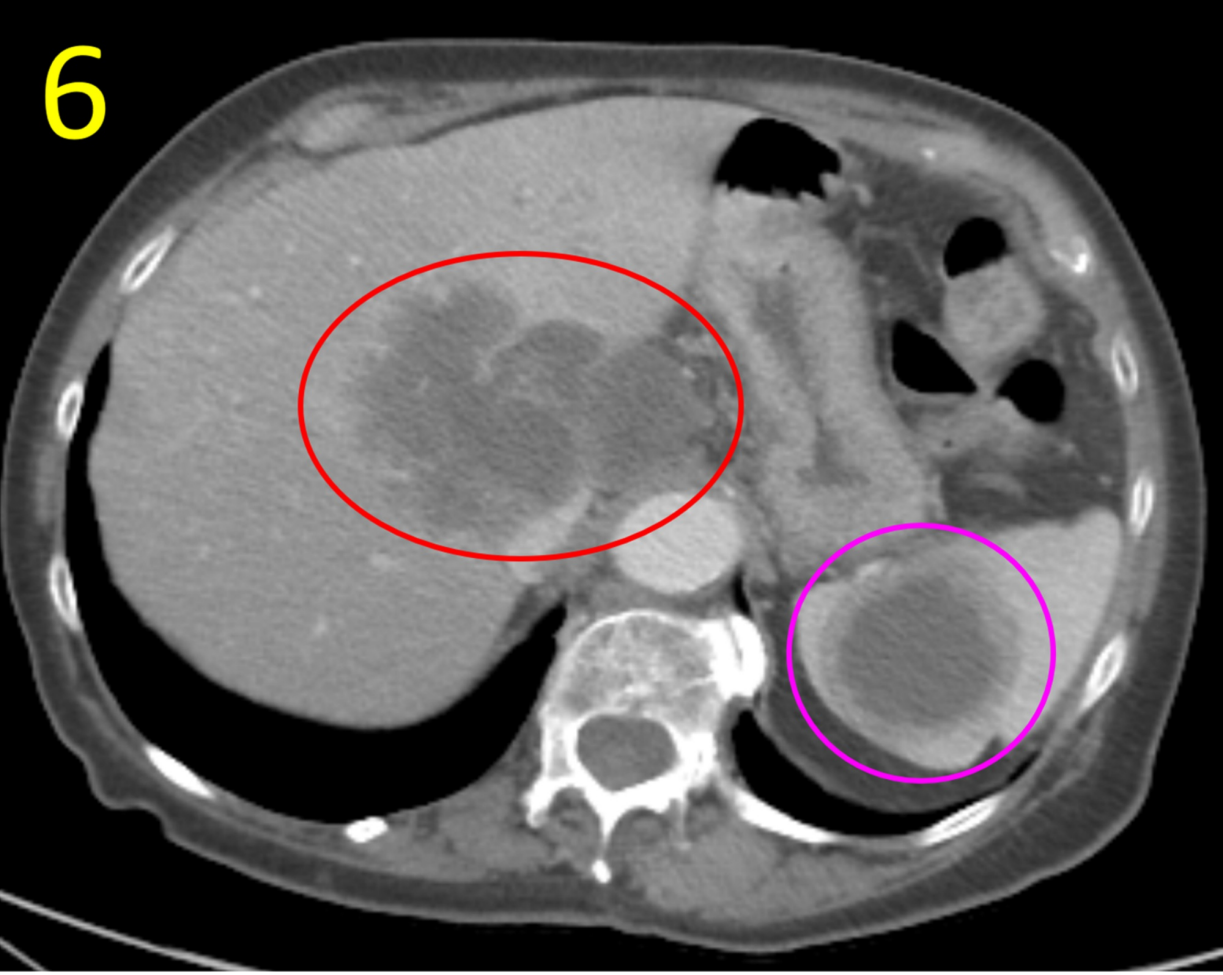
Abdominal CT Scan This abdominal CT scan demonstrates large masses in the liver (red circle) and spleen (magenta circle) that represent likely metastases from the small cell lung carcinoma mass visualized on chest CT.
